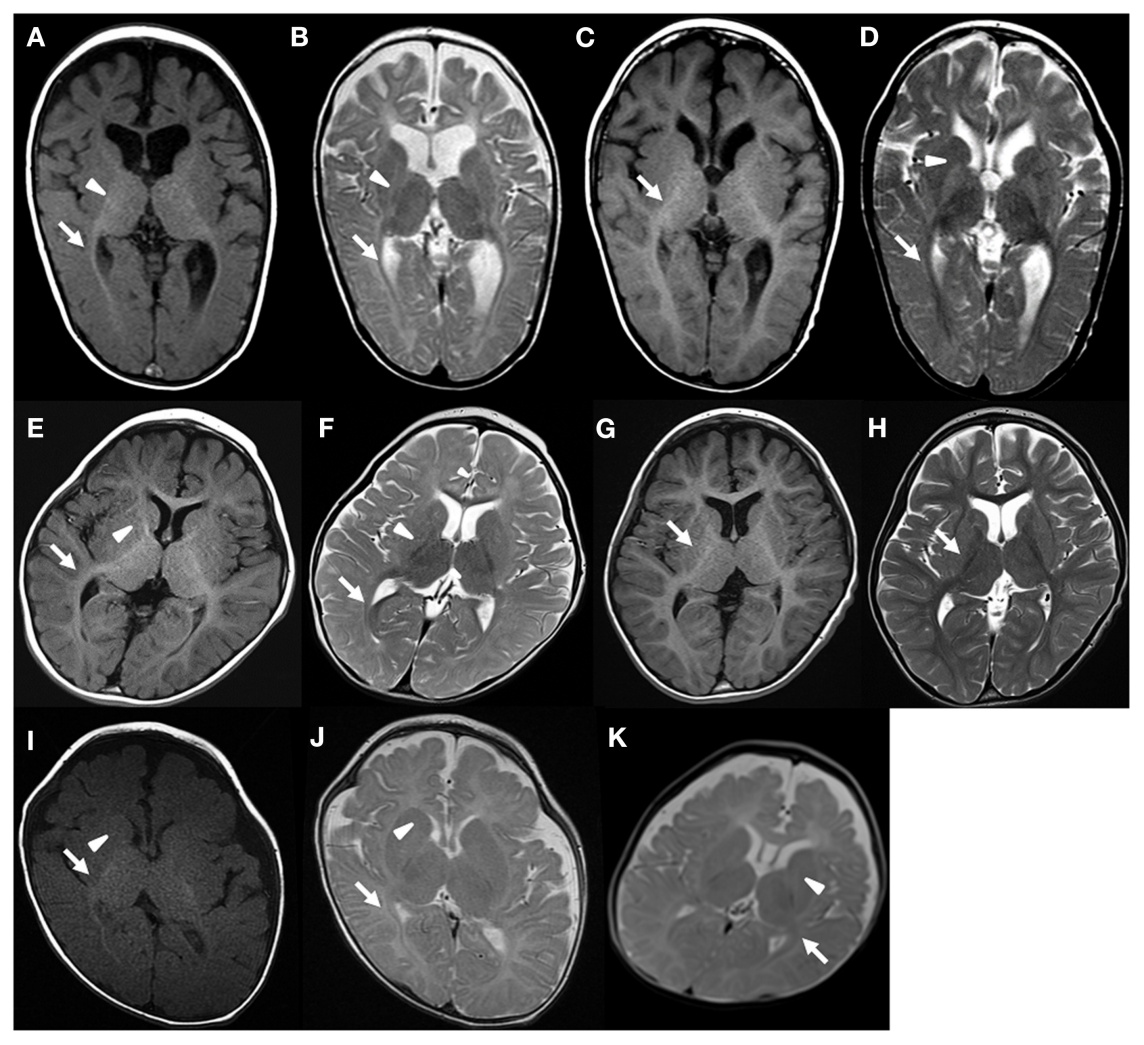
Brain MRI of patient 1 (A,B) 8 months, (C,D) 3 years and 1 months, patient 2 (E,F) 1 year and 4 months, (G,H) 6 years, patient 3 (I,J) 5 months, and patient 4 (K) 6 months. Arrow and arrow head indicates myelination and lack of myelination, respectively. The regional myelination status can be found in Table 1.
